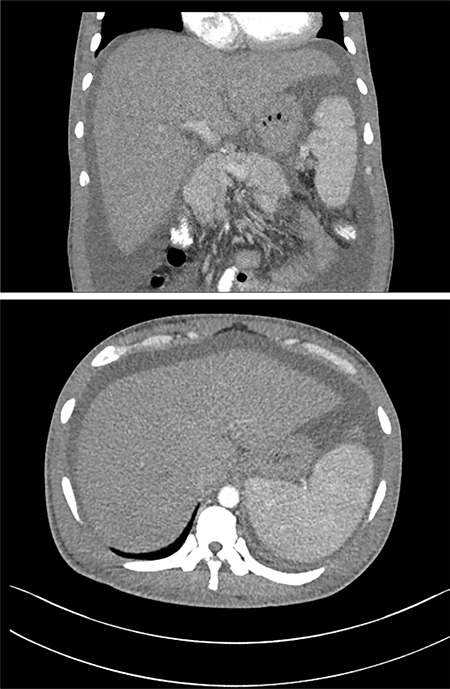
Computed tomography scans of the abdomen showing hepatosplenomegaly
